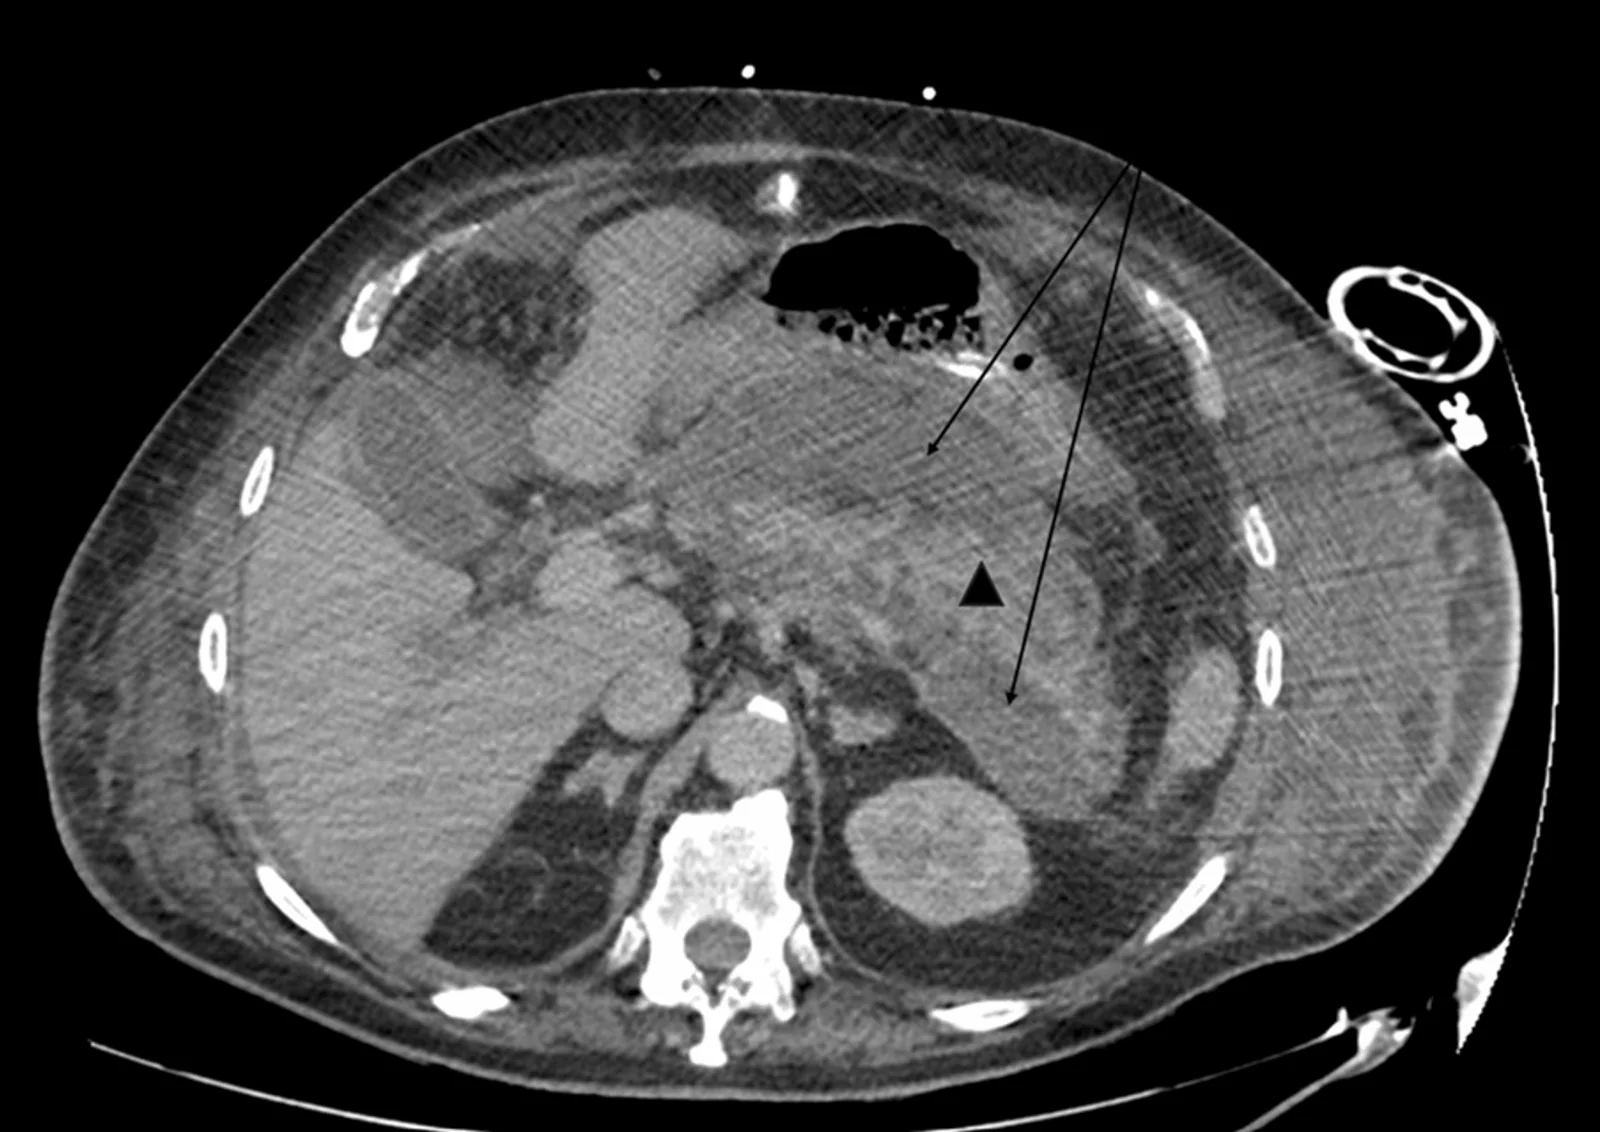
Axial contrast-enhanced abdominal CT scan. The image demonstrates diffuse pancreatic enlargement (arrowhead) with heterogeneous enhancement indicative of necrosis, accompanied by extensive peripancreatic fat stranding and significant fluid collections (arrows) in the peripancreatic and retroperitoneal spaces, consistent with Balthazar stage E classification.
